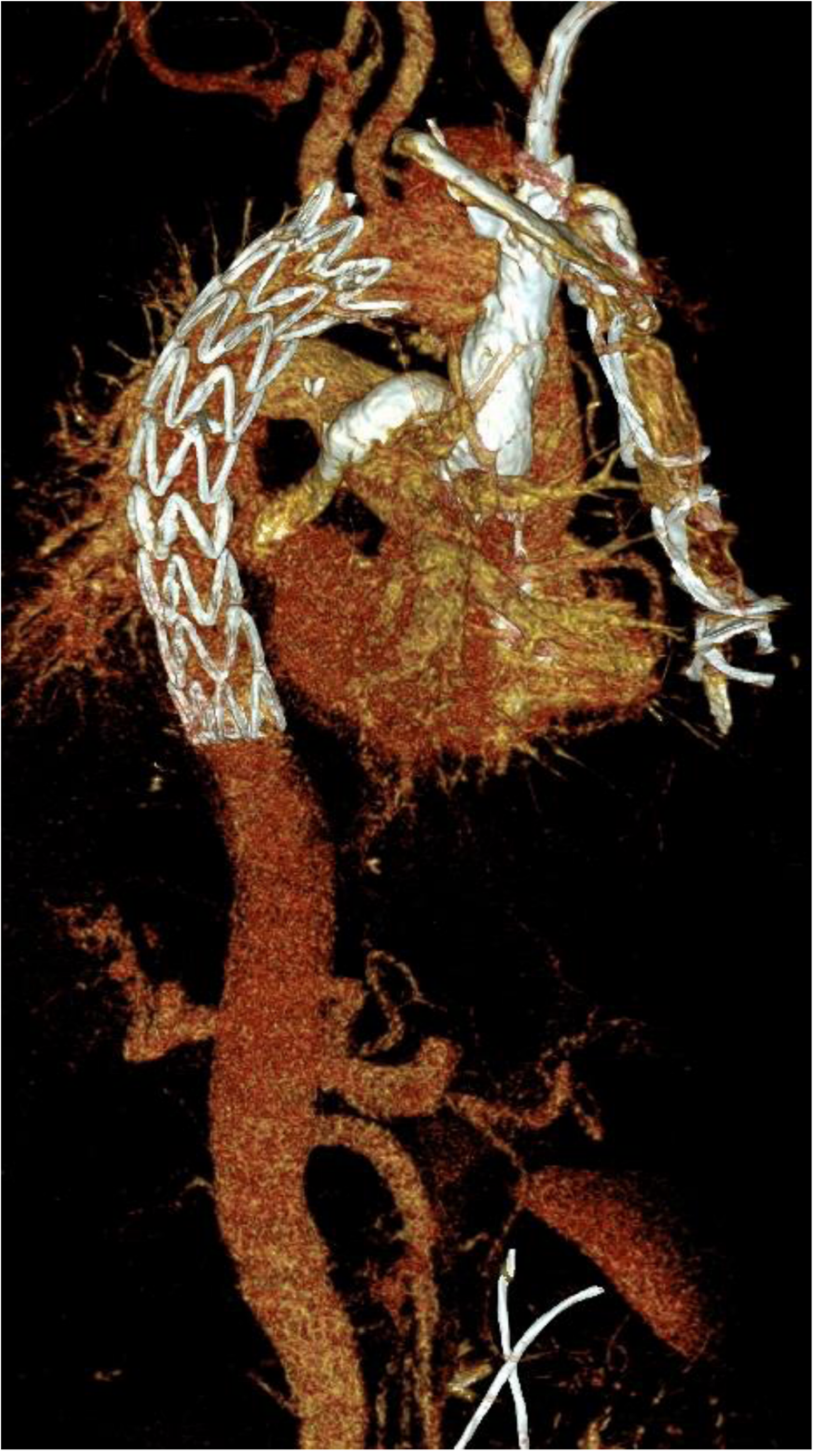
Postinterventional CT scan after deployment of stentgraft
